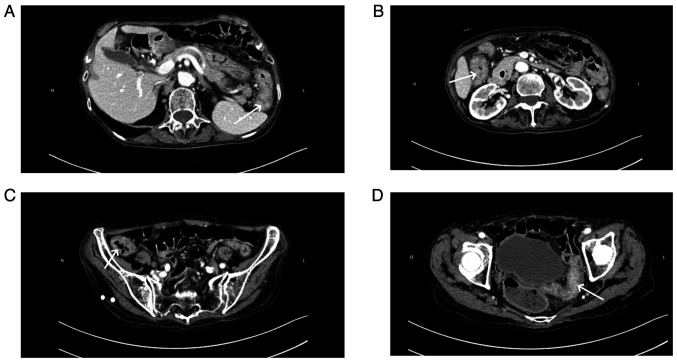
Abdominal contrast-enhanced computed tomography scan images of a patient with involvement of the total colon. (A) A thickened colonic wall (arrow) at the splenic flexure was observed. Edema and a thickened bowel wall (arrows) in the (B and C) ascending colon and (D) sigmoid colon were observed. R, right side of the patient; L, left side of the patient.
